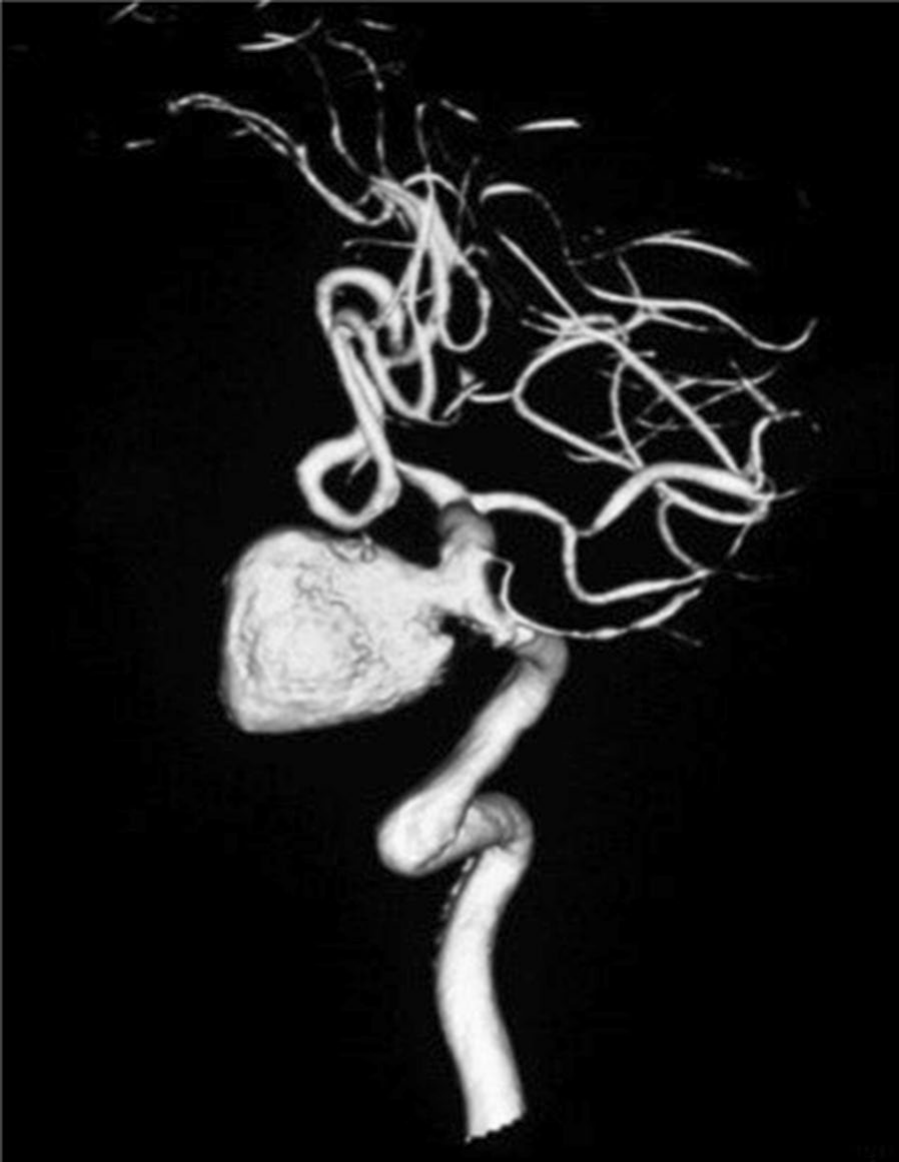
Saccular aneurysm arising from intra-cavernous segment of left internal carotid artery seen in CT angiogram
